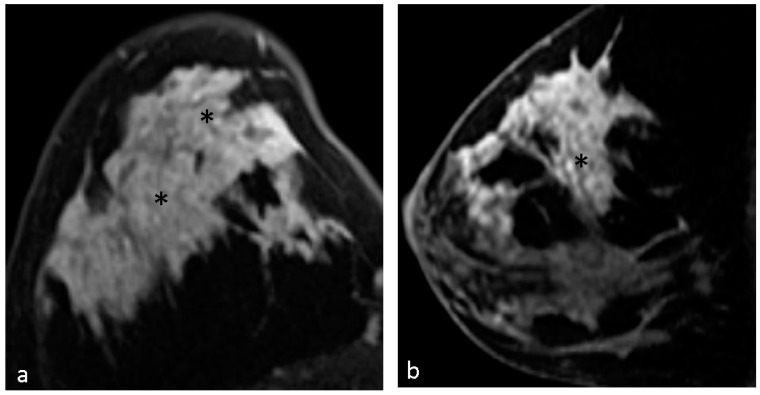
A 36-year-old woman with right breast mass for one month and red nipple discharge. Axial (a) and sagittal (b) T1-weighted contrast-enhanced MR imaging shows clustered ring NME lesion (*) in right breast with multifocal distribution. Pathological results of right breast revealed infiltrating duct carcinoma and ductal carcinoma in situ.
